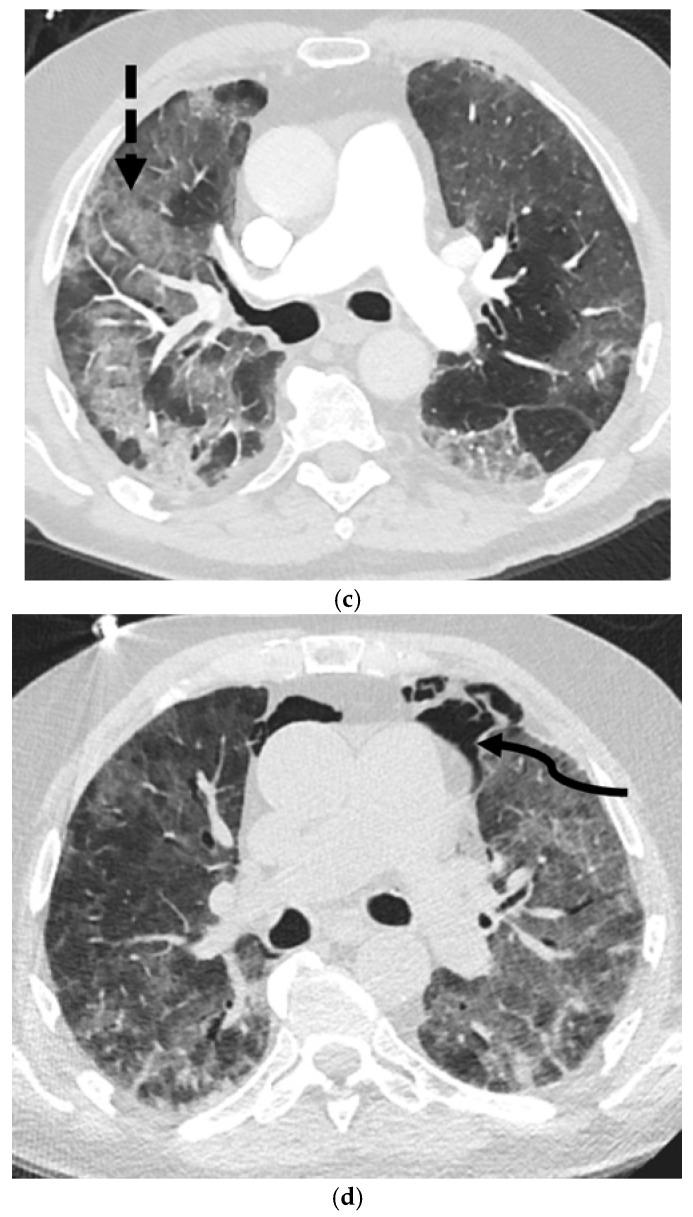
77-year-old male with a history of mantle cell lymphoma, recently hospitalized for COVID-19 pneumonia, now re-admitted 1 week later with persistent hypoxemic respiratory failure. Axial CT image in lung window at baseline (a) demonstrates peripheral predominant ground-glass opacities (dashed black arrow) in lungs consistent with known COVID-19 pneumonia. Repeat axial CT images in lung window obtained 1 week later (b–d) demonstrate worsening ground-glass opacities (dashed black arrow) in lungs along with a small new left pneumothorax (open white arrow) and pneumomediastinum (curved black arrow).
